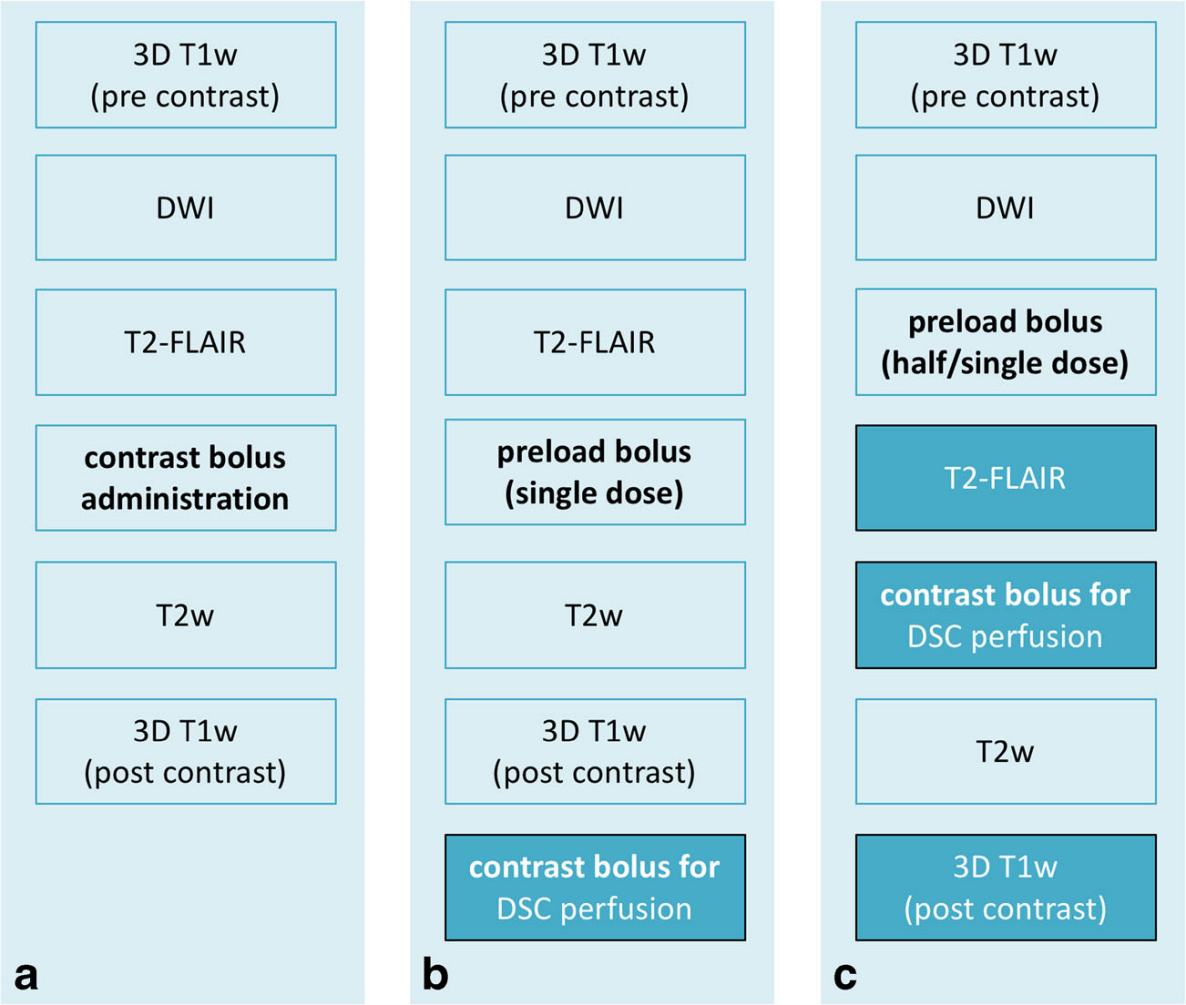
Three possible options for a glioma imaging protocol in clinical practice based on the EORTC-NBTS protocol (a), with the addition of DSC perfusion imaging (b, c). Option C has the advantage over option B that it has double the contrast dose for post-contrast T1w imaging. Option B may be preferred if non-contrast enhanced T2-FLAIR is desired. Please see Ellingson et al. [9] for further considerations and vendor-specific sequence details on structural and diffusion-weighted imaging. The moment of contrast administration is indicated in bold
